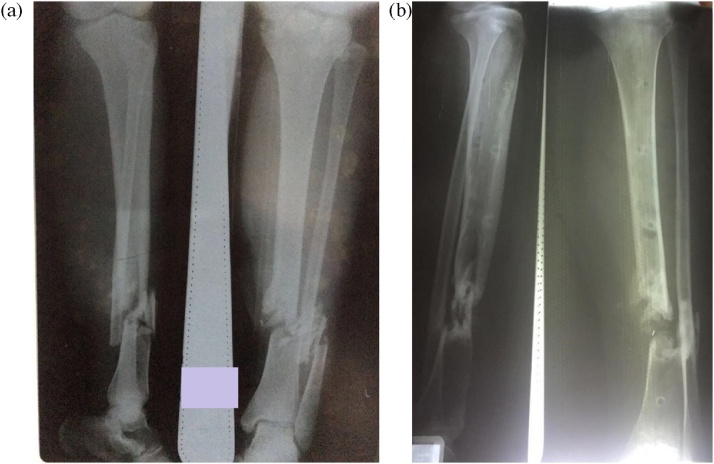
(a) Initial x-ray after the accident, (b) x-ray was taken in the first visit to our hospital with the cast removed (source: internal documentation).
